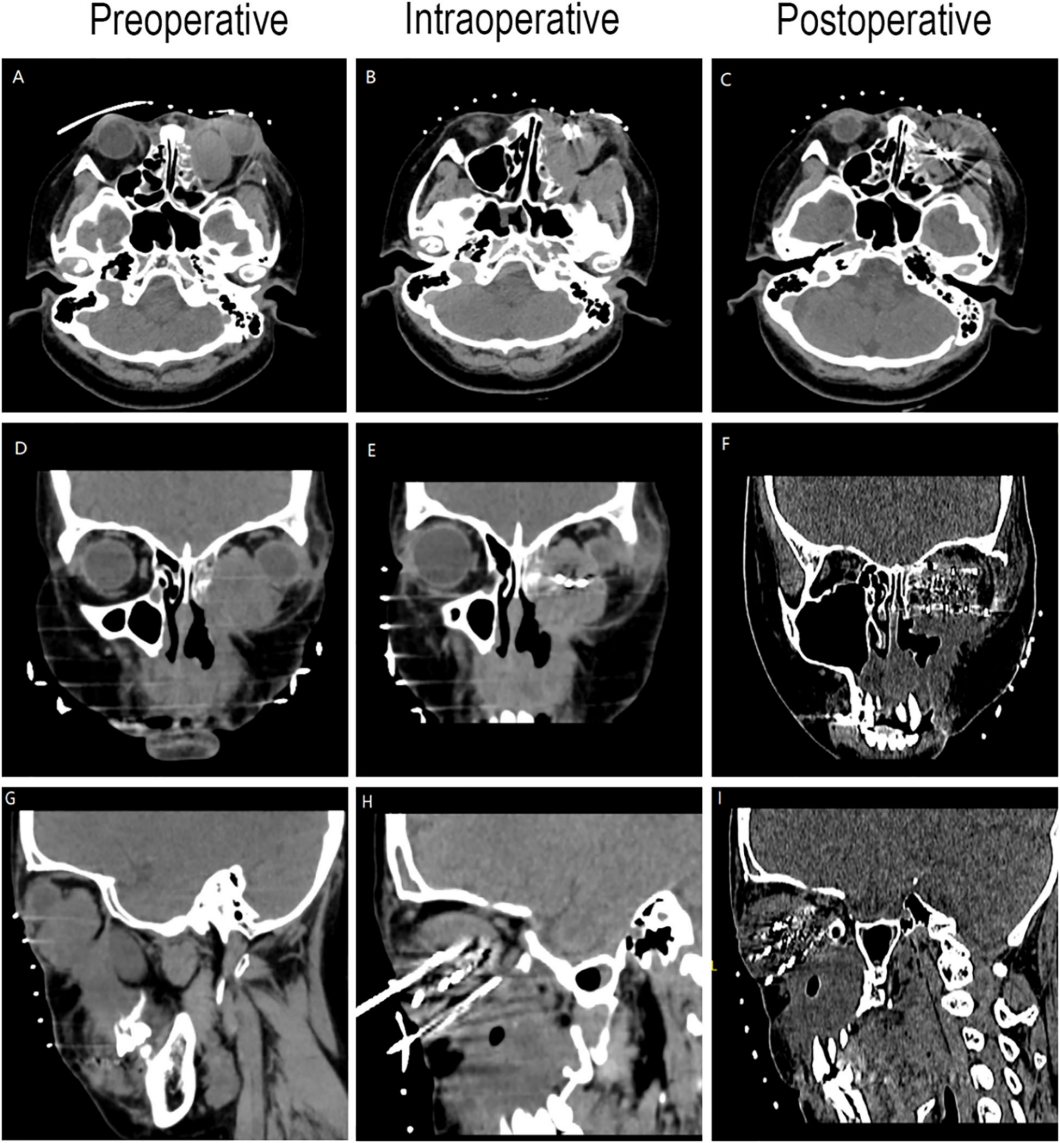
CT-guided iodine-125 seed implantation. (A–C) Axial CT image. (D–F) Coronal CT image. (G–I) Sagittal CT image.
